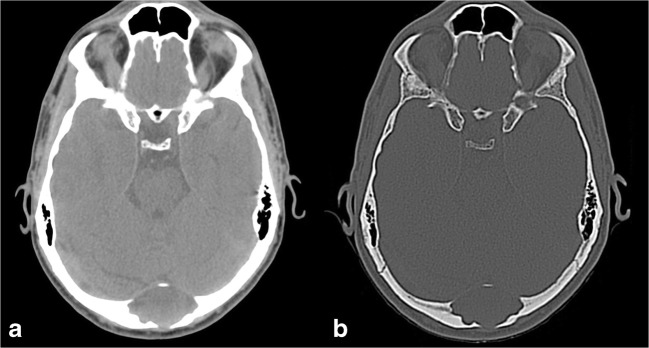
Intradiploic epidermoid cyst (IEC). Axial CT images with soft tissues (a) and bone (b) windows show an occipital hypodense osteolytic lesion with smooth margins and greater involvement of the inner table, extensively demineralised
